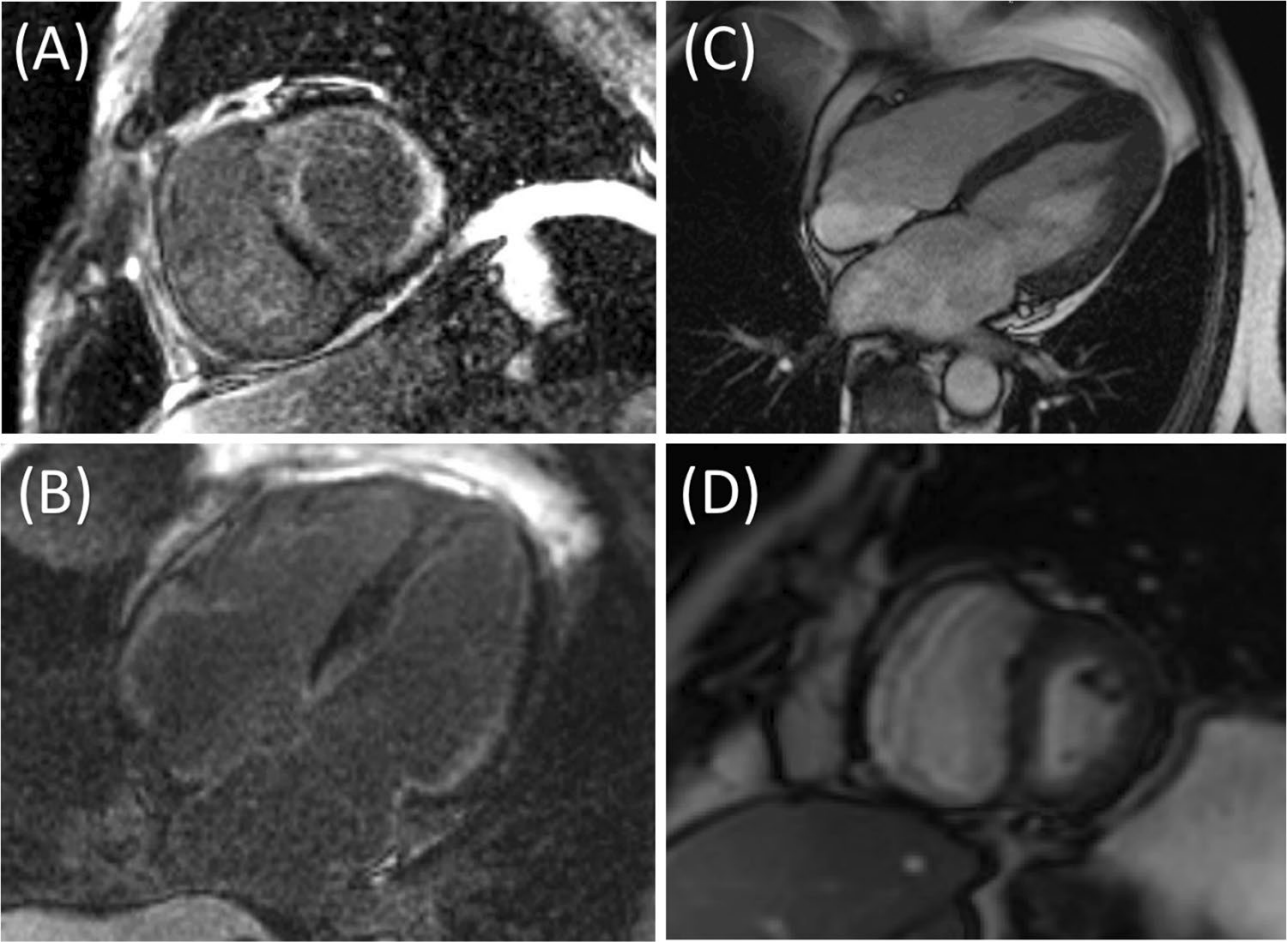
Specific causes of heart failure with preserved ejection fraction (HFpEF) diagnosed on cardiac magnetic resonance imaging (CMR). a Short axis late-gadolinium enhancement (LGE) sequence in a patient with HFpEF secondary to cardiac amyloidosis. Diffuse subendocardial enhancement is seen in the left and right ventricles. b Apical 4-chamber view in the same patient showing subendocardial LGE. c Apical 4-chamber cine in a patient initially diagnosed with ‘HFpEF’. Increased wall thickening and hypertrophy are seen at the left ventricular (LV) apex typical of apical hypertrophic cardiomyopathy. d Short axis cine in a patient presenting with ‘HFpEF’, demonstrating pericardial thickening and ‘D’ shaped flattening of the septum towards the LV during diastole. Subsequently diagnosed with pericardial constriction
